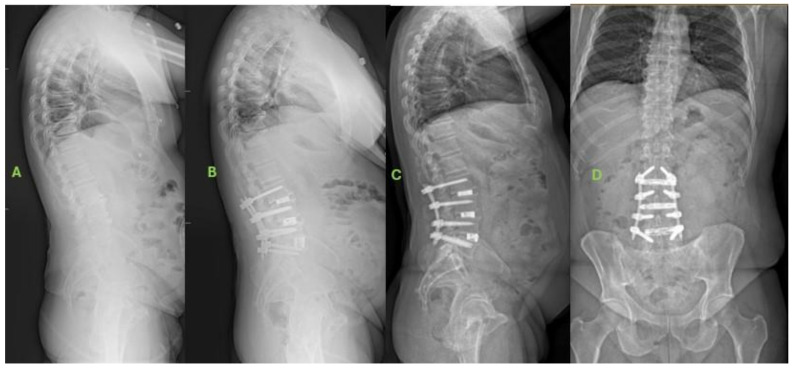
Example of SIJP− patient. (A) Baseline radiographs of 65-year-old male with preoperative diagnoses of loss of lumbar lordosis, lumbar spondylosis, bilateral radiculopathy, and spinal stenosis. (B) Six-week postoperative radiographs. The patient underwent L2-L5 lateral lumbar interbody fusion and L2-S1 posterior spinal fusion. (C,D) Two-year anteroposterior and lateral radiographs. Significant improvement in preoperative symptoms with maintained alignment still demonstrated at 2 years.
